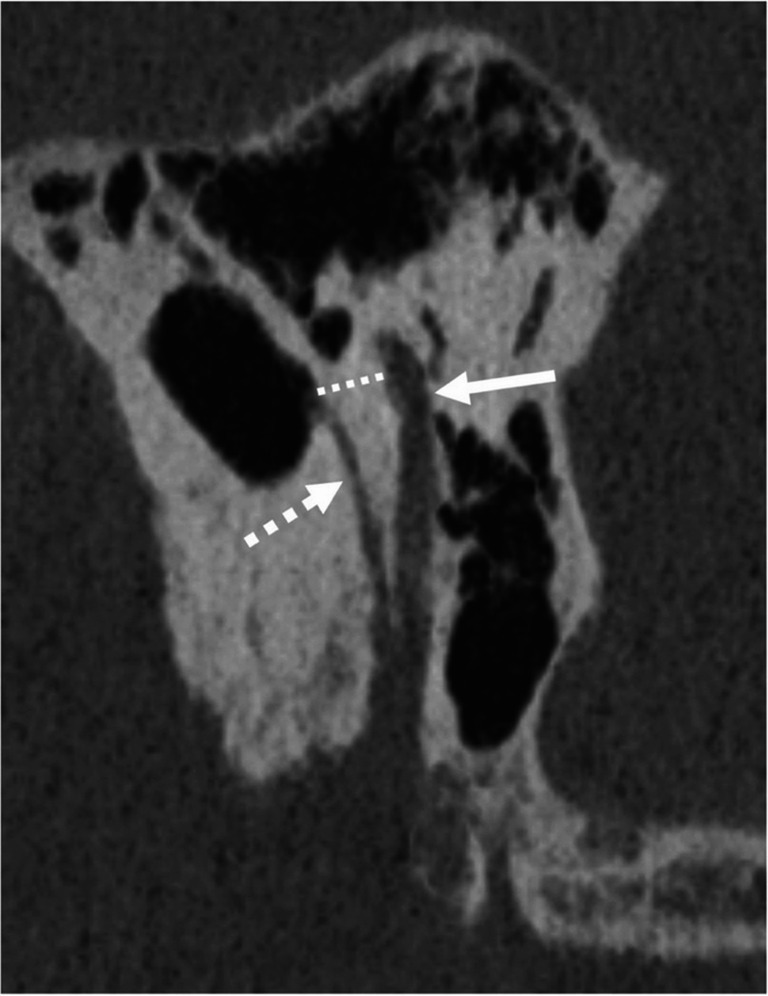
Parasagittal measurement of facial recess width (FRW). Oblique-sagittal reformatted CT demonstrating FRW (dashed lines) as the perpendicular distance between the mastoid facial nerve canal (solid arrows) and the chorda tympani (dashed arrows) at the level of the widest portion of the facial recess
